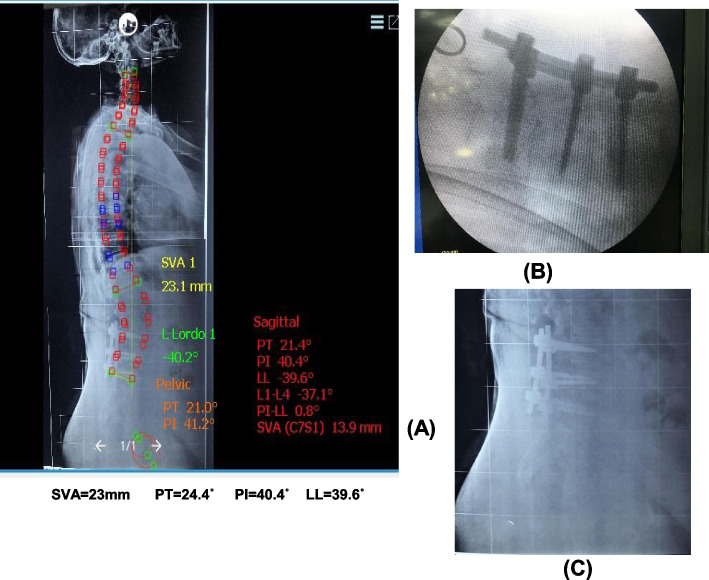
A Postoperative X-ray of whole spine shows postoperative parameters of SVA and pelvic parameters. B Intraoperative imaging of instrumentation. C Lateral X-ray of lumbar spine postoperative
